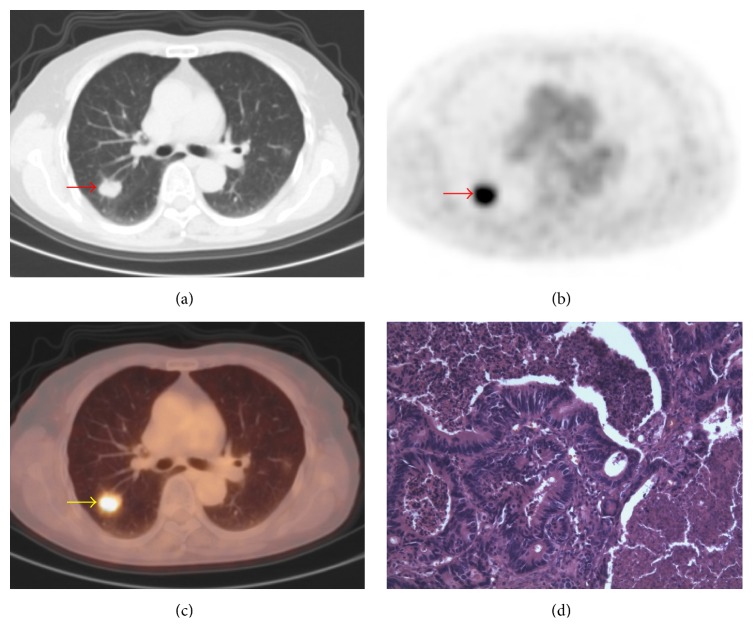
A false-negative PET/CT finding in a 64-year-old female patient. (a) On CT image, the nodule in the right upper lobe with lobulation and low density is observed (arrow); the maximum diameter of nodule is 17 mm. (b) On PET and (c) on PET/CT image, increased ^18^F-FDG uptake is detected; SUV_max_ = 7.4; the result of PET imaging is infection. (d) Pathologic examination suggests metastatic adenocarcinoma. The *y* value calculated by our model is 0.73. The result of our model is consistent with PET and pathological result but inconsistent with PET/CT.
